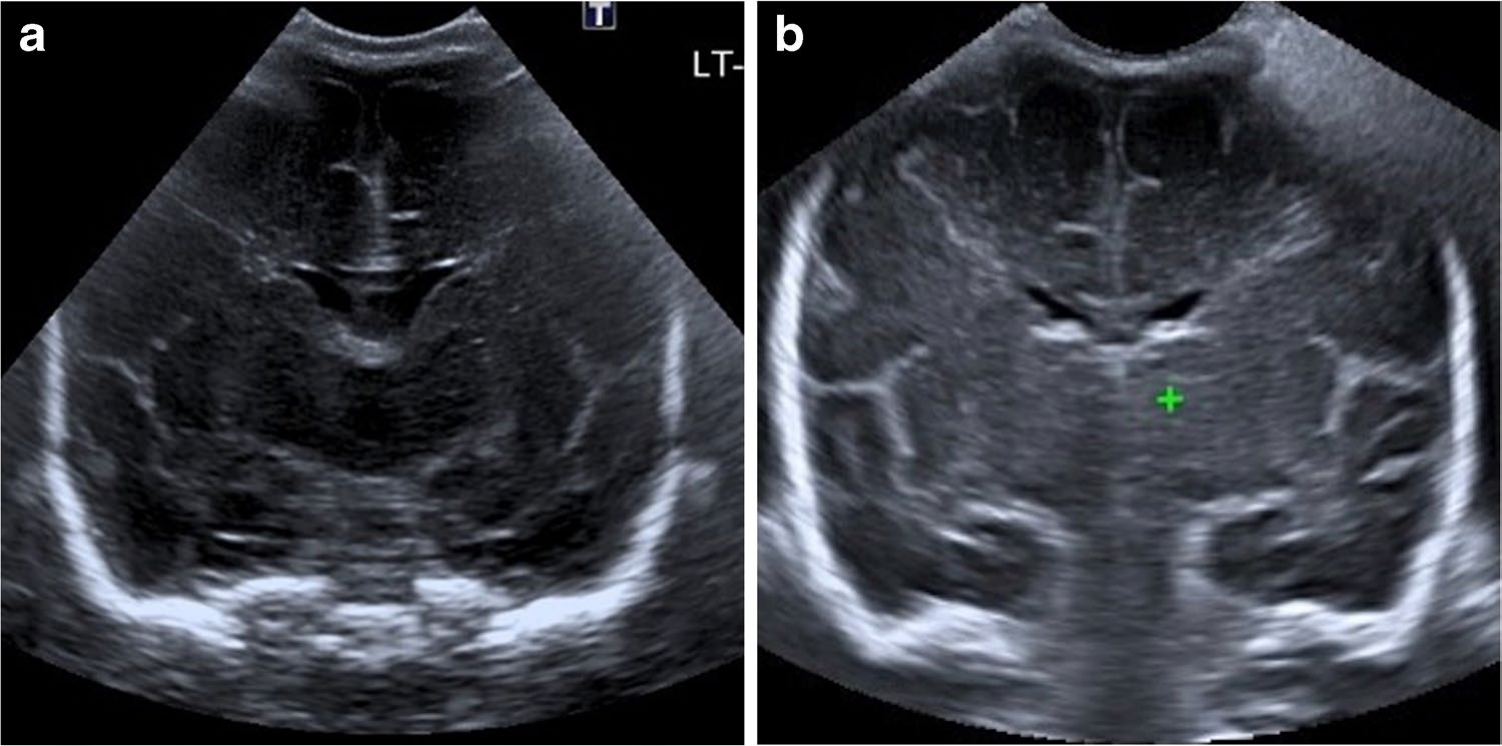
A 25-day-old girl with a normal cranial ultrasound (US). a Two-dimensional (D) US coronal view of the temporal uncus and Sylvian fissure. All three observers rated this view quality ‘adequate’. b Coronal reconstruction of the 3-D volumetric US dataset of the temporal uncus and Sylvian fissure view. Two observers rated this view quality ‘excellent’, and one ‘good’
